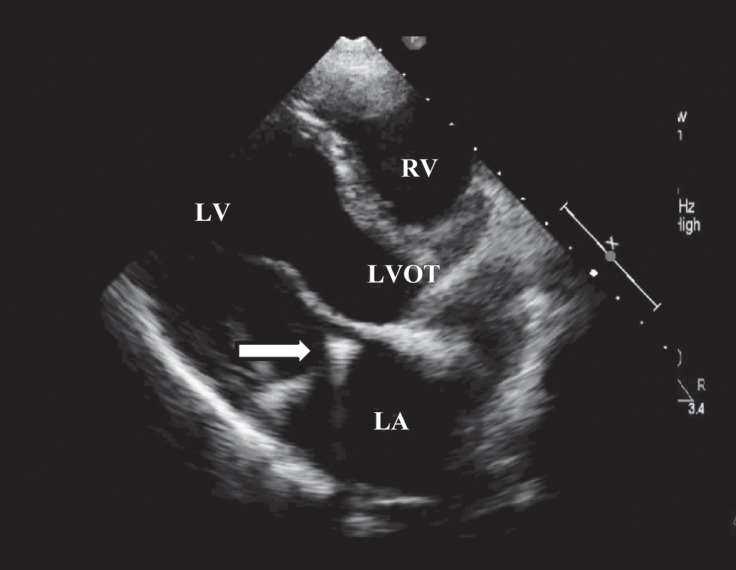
Parasternal long-axis view of the echocardiogram, showing gross dehiscence in the mitral ring (arrow).
